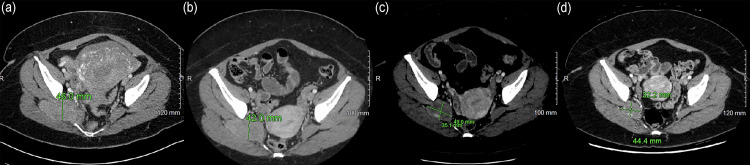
Abdominal and pelvic CT demonstrating (a) initial pelvic mass affecting the right sciatic nerve, (b) post-cycle 3, (c) post-cycle 6, and (d) post-cycle 12 changes to the pelvic mass.
